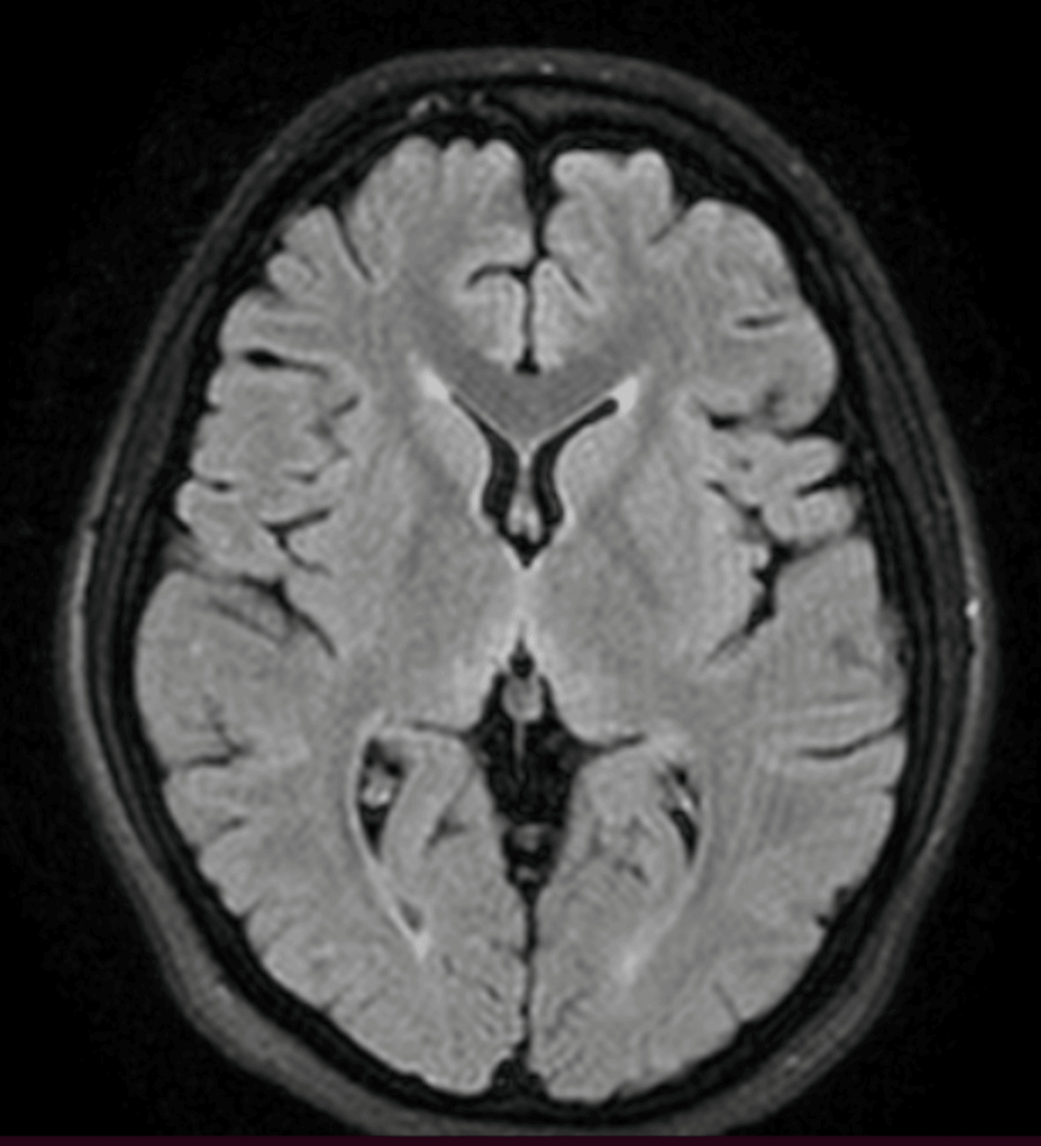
Axial T2 Fluid-Attenuated Inversion Recovery (FLAIR) MRI images of the brain show normal signal intensity throughout, with no evidence of abnormal hyperintensities or lesions
